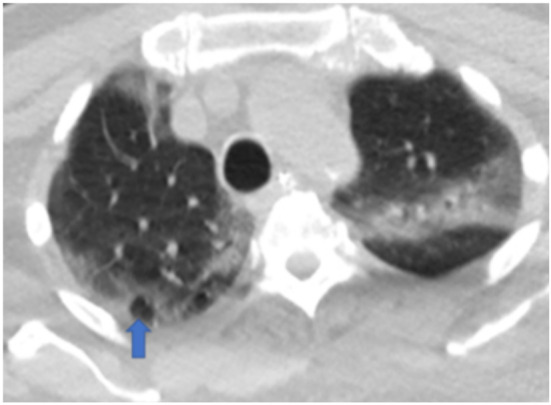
An axial non-enhanced chest CT scan, lung window of a 76-year-old man with a 5-day history of fever shows right peripheral ground glass infiltrates and cavitation (Arrow). Consolidation on the left. RT-PCR test was positive.
